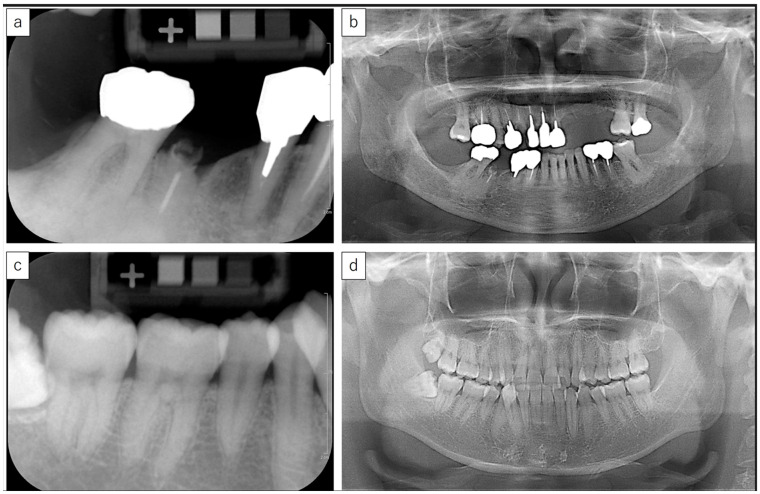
Representative intraoral and panoramic radiographs of osteoporotic and non-osteoporotic cases. Representative intraoral radiograph and panoramic images of an osteoporotic case (a,b) and non-osteoporotic case (c,d). Note the sparse trabecular structure and cortical erosion in (a,b) compared with the denser trabecular pattern and intact cortex in (c,d).
